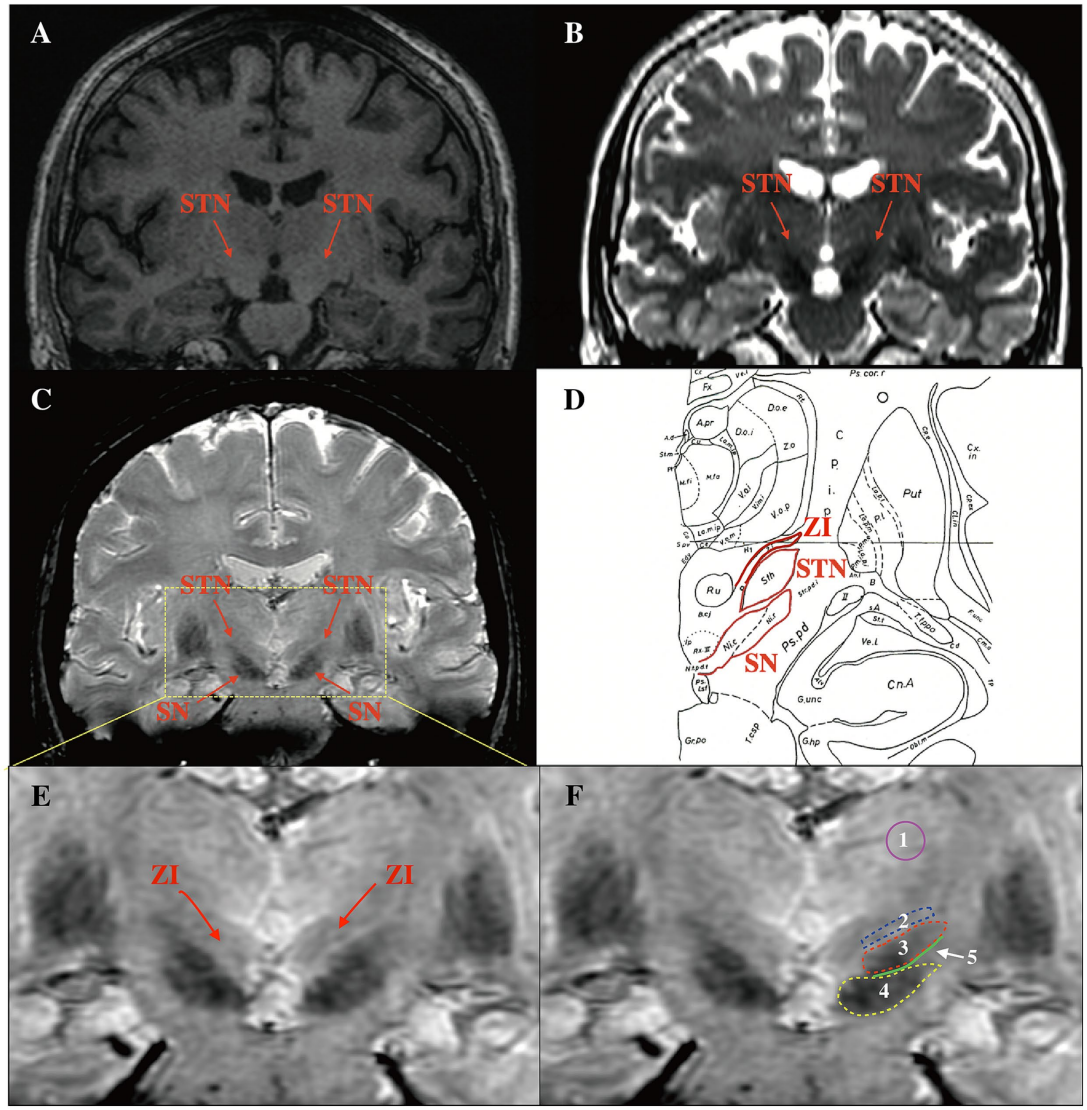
Comparison of the delineation of the STN in different coronal sequences and ROI selection on SWI. (A) The STN could not be visualized on T1w. (B) The STN and SN were hypo-intense on T2w, but the dorsoventral borders of the STN were difficult to distinguish. (C) The STN was hypo-intense on SWI, and the dorsoventral borders were visible. (D) Use of the Schalterbrand atlas (plate 27 Fp 3.0) to display relationships between the STN and surrounding structures. (E) The ZI was visible on SWI. (F) ROI selection. 1: thalamus area 2: ZI 3: STN 4: SN 5: the junction area between the STN and the SN. STN, subthalamic nucleus; ROI, regions of interest; SWI, susceptibility weighted imaging; SN, substantia nigra; ZI, zona incerta; T1w, T1-weighted; T2w, T2-weighted.
